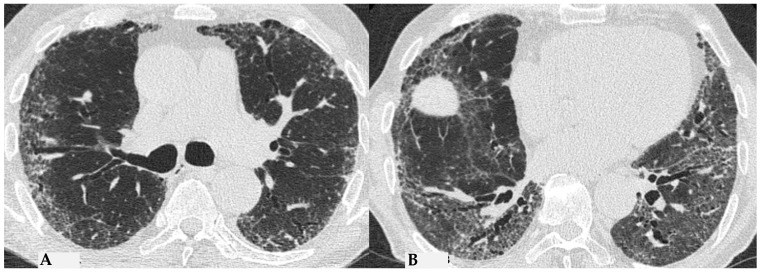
f-NSIP pattern: chest HRCT, axial scan. Mild fibrotic changes of limited extent with a greater distribution in the upper lobes with superimposed GGO (A); the lower lobes show marked extension of GGO, fine reticulations with traction bronchiectasis/bronchiolectasis (B); “fibrotic NSIP pattern” according to new HP guidelines (2020) [4].
